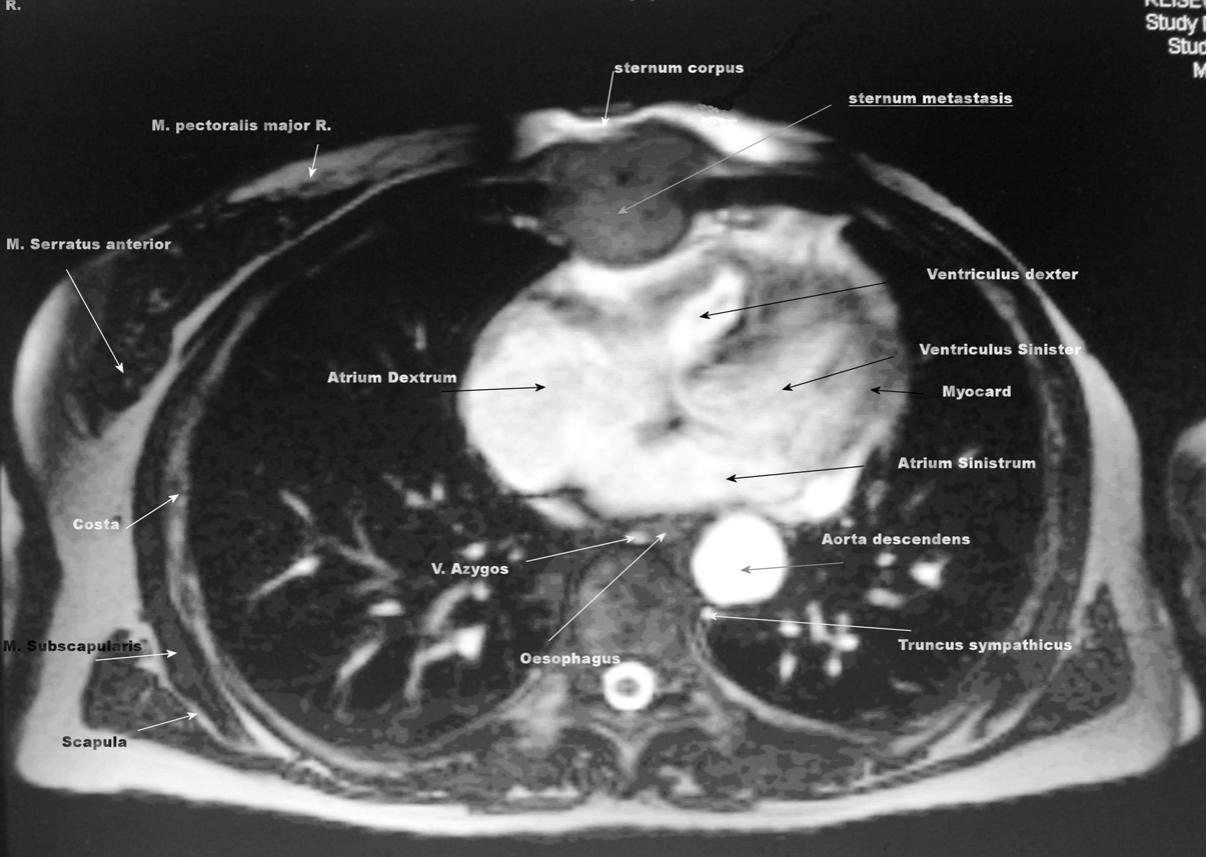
Magnetic resonance imaging of axial plan with intravenous contrast gadolinium-BOPTA demonstrating a mass adherent to the right ventricular wall.
